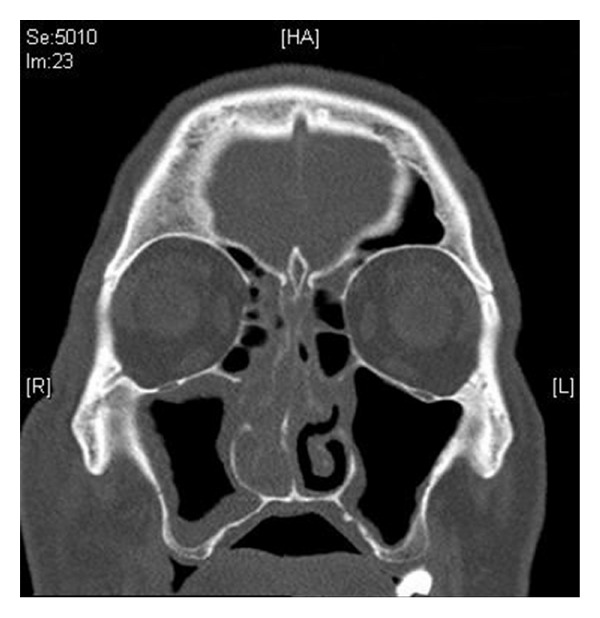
Coronal CT view of sinuses showing right-sided sinusitis with mucosal thickening of the maxillary sinus and anterior ethmoids.
